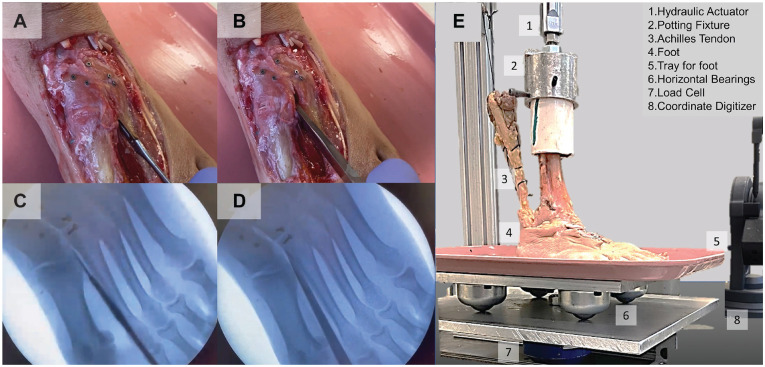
The creation of the purely ligamentous isolated Lisfranc injury using (A) a 10-blade scalpel to carefully section the dorsal, interosseous, and plantar Lisfranc ligaments and then (B) a narrow osteotome to widen the bones while under load. (C) The scalpel and (D) the osteotome were visualized under radiographic guidance to prevent disruption to any other ligamentous structure in the midfoot. (E) The loading apparatus with a numbered legend.
